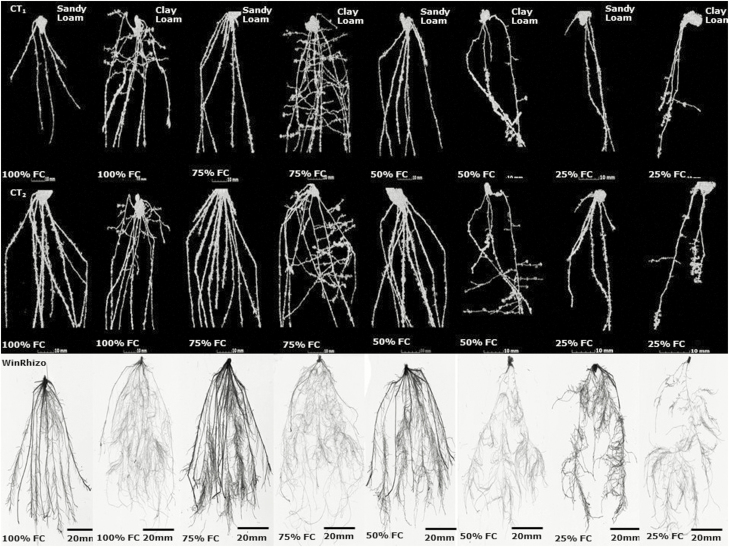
CT images of the roots of Zebedee 14 (CT_1_) and 21 (CT_2_) days after germination grown under water deficit (100, 75, 50 and 25 % FC) in the sandy loam and the clay loam soils and root systems (at 21 days) of the same plants after extraction and analysis using WinRHIZO® equipment compared to CT_2_ images. Scale bars represent 10 and 20 mm.
